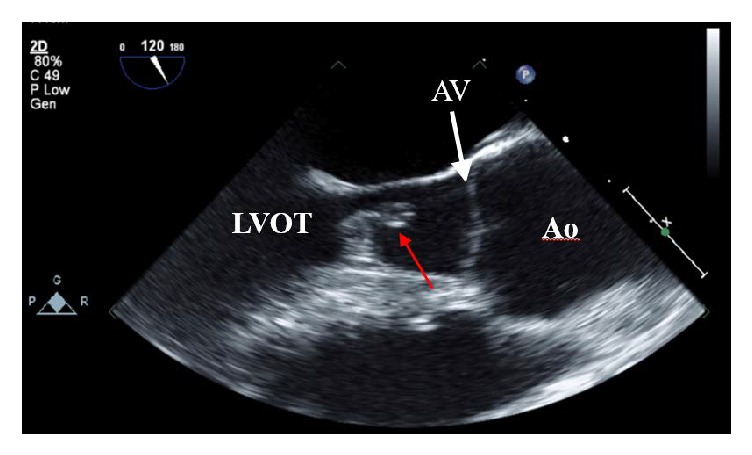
Transesophageal echocardiogram mid-esophageal position demonstrating a 1.3 by 1.0 cm mass (red arrow) attached to the interventricular septum in the left ventricular outflow tract during diastole. LVOT = left ventricular outflow tract, Ao = aorta, and AV = aortic valve.
